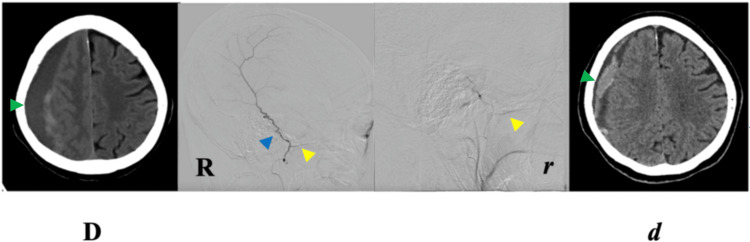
Case D. Pre-EMMA (D) and respective post-EMMA (d) cranial NCCT axial views at levels of maximum CSDH thickness. Pre-EMMA angiography in right lateral projection (R) shows the course of posterior MMA branch and absence of anterior MMA branch, and post-EMMA obliteration of the posterior branch (r). Angiographic projections correspond to contiguous NCCTs. EMMA, embolization of the middle meningeal artery; MMA, middle meningeal artery; NCCT, non-contrast computed tomography; R, right. Green arrowhead, chronic subdural hematoma; blue arrowhead, posterior branch; yellow arrowhead, sphenoidal branch.
